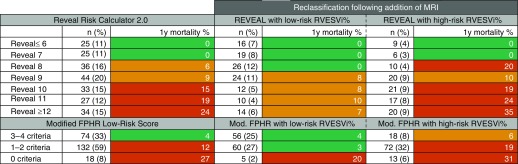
Impact of adding cardiac magnetic resonance imaging to REVEAL 2.0 and a modified FPHR (French Pulmonary Hypertension Registry) approach to European Society of Cardiology and European Respiratory Society guidelines for risk stratification. Green indicates low risk of 1-year mortality (<5%), amber indicates intermediate risk (5–10%), and red indicates high risk (>10%). Mod. = modified; MRI = magnetic resonance imaging; PAH = pulmonary arterial hypertension; REVEAL = North American Registry to Evaluate Early and Long-Term PAH Disease Management; RVESVi% = percentage-predicted right ventricular end-systolic volume index.
